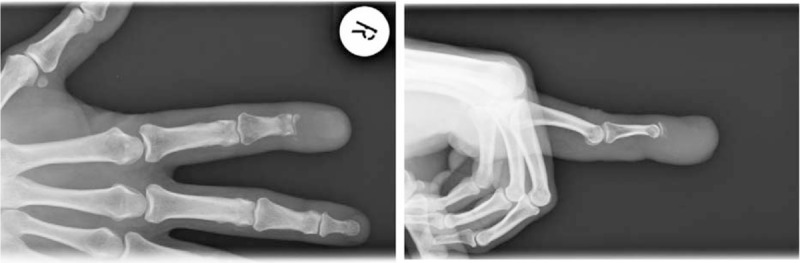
Hand X-ray: PA and lateral view.
